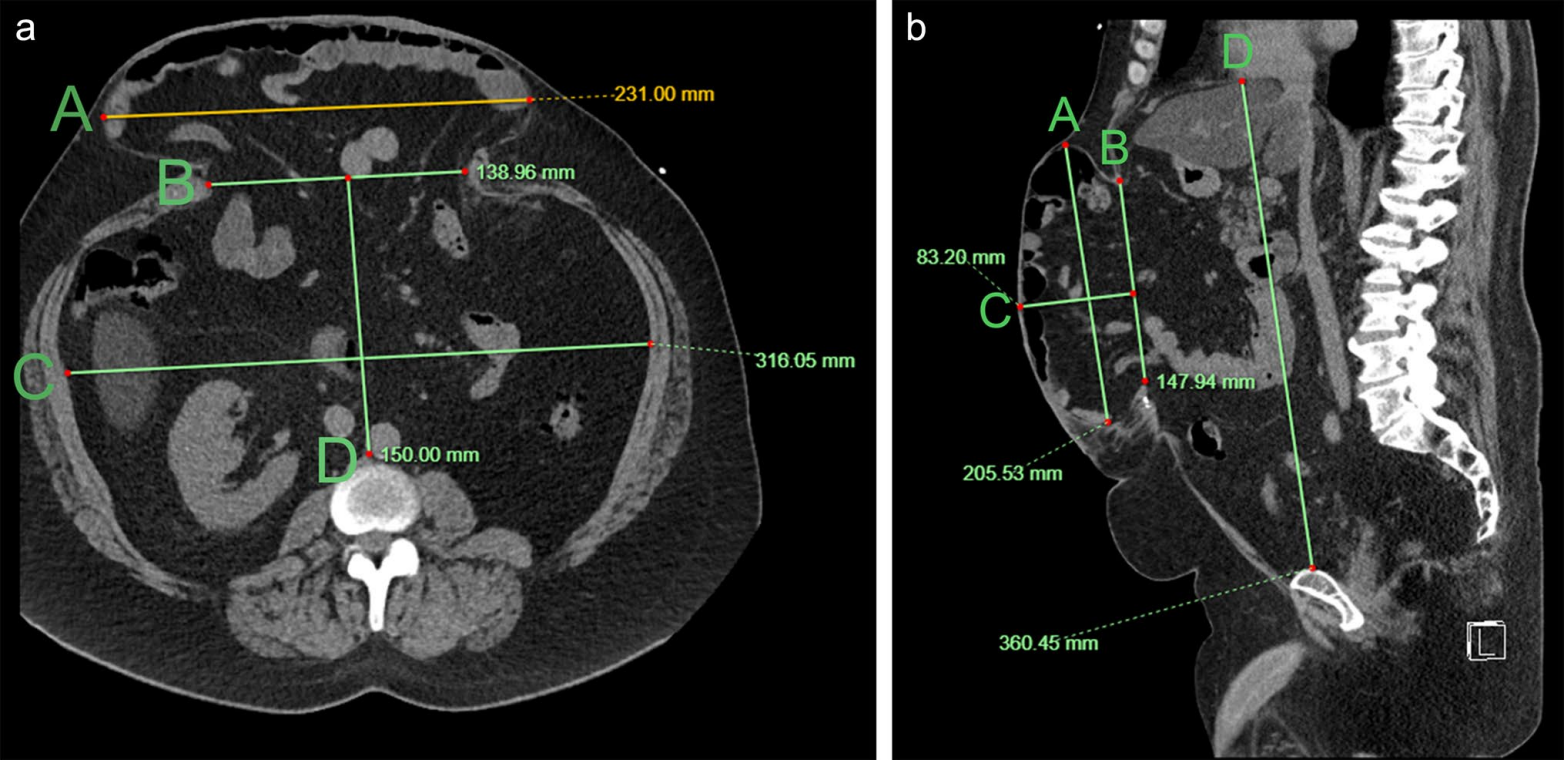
Non-contrast-enhanced abdominal computed tomography denoting measurements of abdominal and hernia cavities dimensions in axial (a) and sagittal (b) projections. On the axial projection (a), line A denotes the widest axial diameter of the hernia sac, line B is measured at the hernia neck, and lines C and D denote the widest transverse and anteroposterior abdominal cavity dimensions, respectively. On the sagittal projection (b), line A denotes the widest craniocaudal dimension of the hernia sac, line B denotes the widest craniocaudal dimension of the hernia neck, line C denotes the widest anteroposterior dimension of the hernia sac, and line D is measuring the widest craniocaudal dimension of the abdominal cavity from the diaphragm to the superior aspect of the pubis
